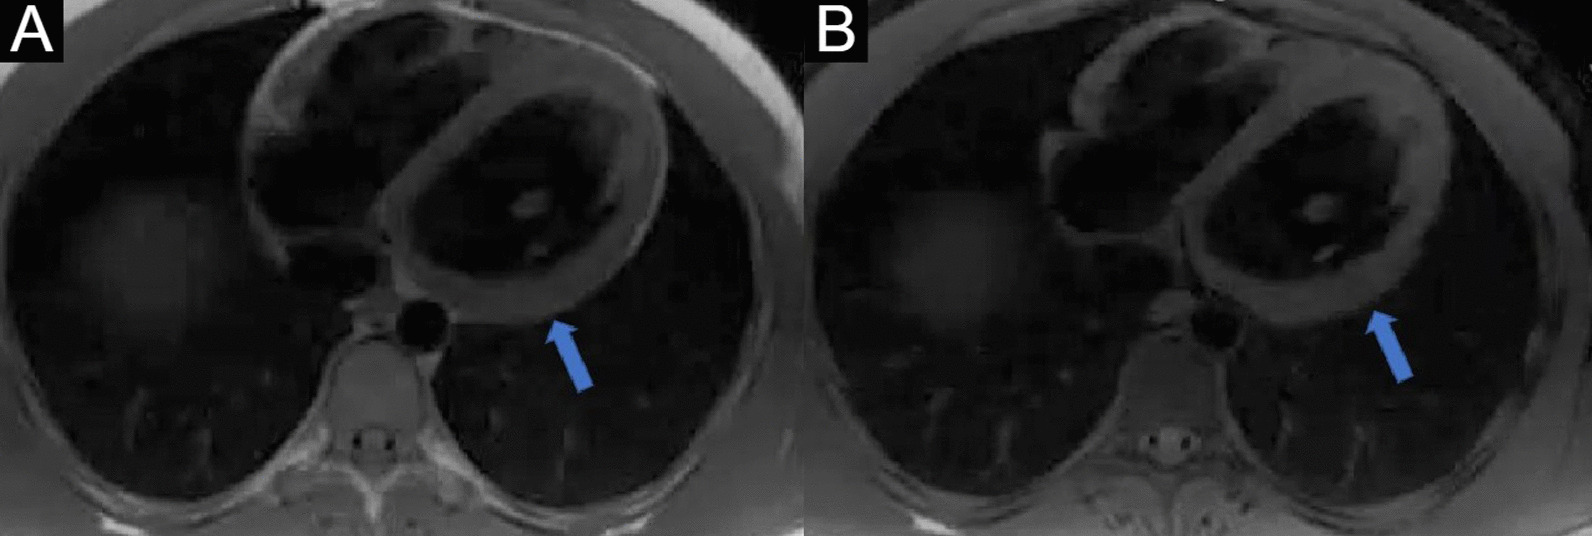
Case 3. Figure 2. Black blood T1-weighted imaging without A and with B fat saturation. Possible epicardial fat infiltration of the lateral LV (arrows)
